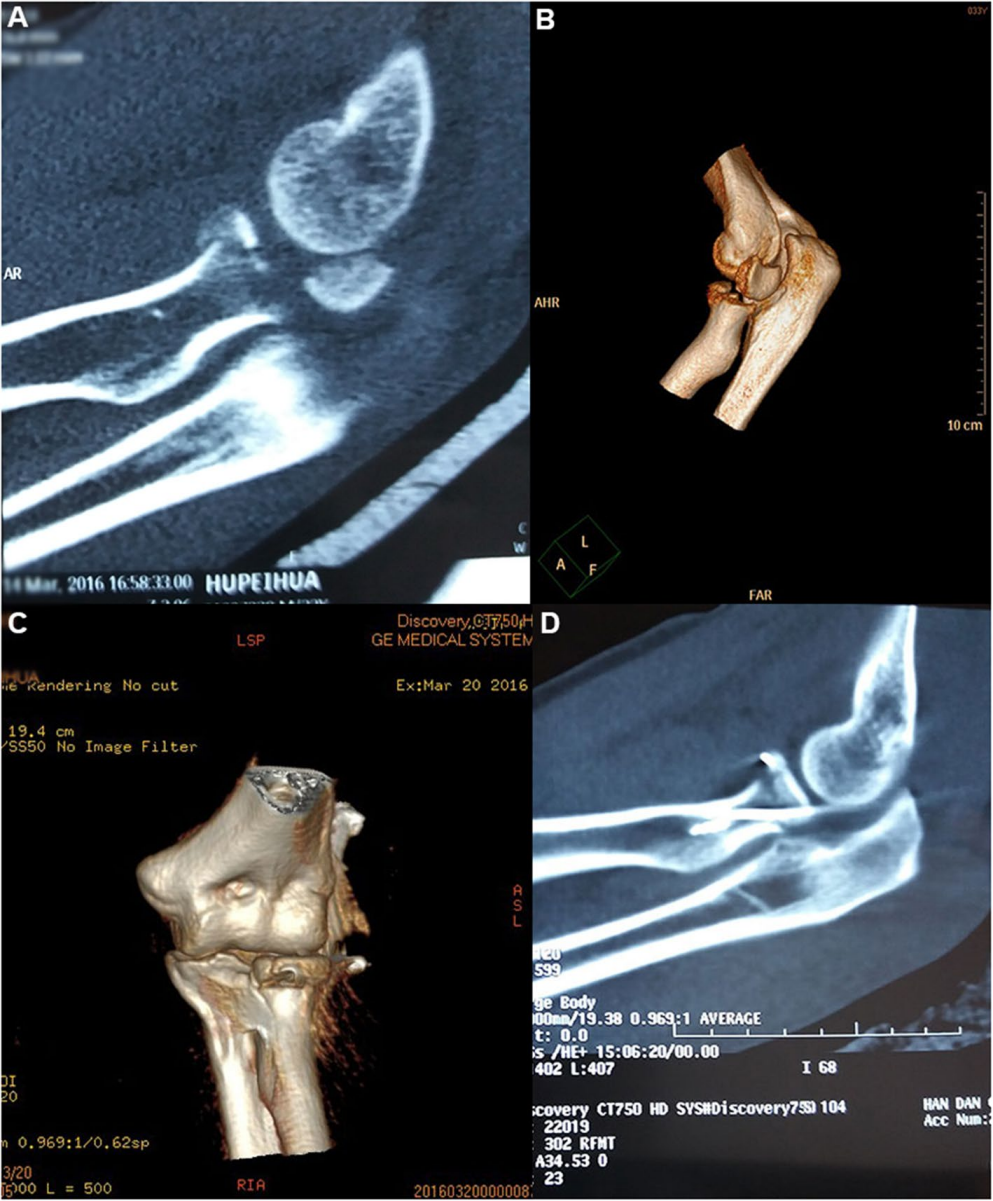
Case 3: A preoperative spiral CT scan reveals a comminuted fracture of the radial head with overturned dislocation (type III) (A and B). A postoperative spiral CT scan reveals that the reduction of the radial head fracture is good (C and D)
